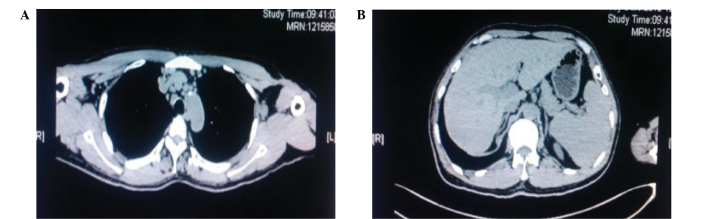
Computed tomography scans showing multiple (A) mediastinal and (B) peritoneal lymphadenopathies.
